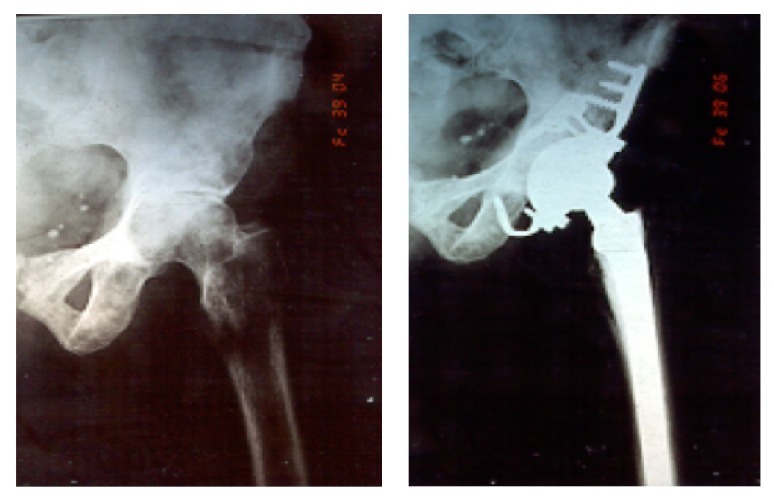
Pre- and postoperative radiographies for Harrington Class 2 bone defect.
